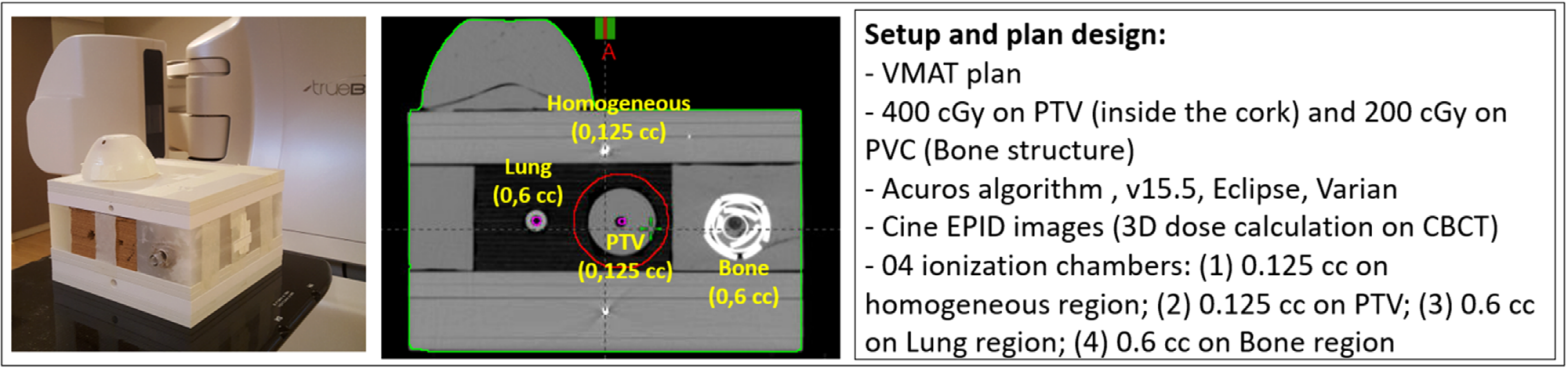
Setup used during the end‐to‐end test to compare doses measured using four ionization chambers with mean doses calculated on sensitive volumes of the detectors by PerFRACTION. EPILOG method was used to calculate the dose on CBCT image.
